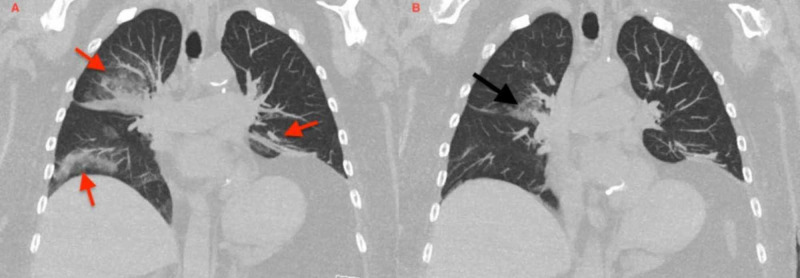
Follow-up CT Chest at three months (panel A) showed partial improvement of bilateral consolidative opacities (red arrows). Follow-up CT at six months (panel B) showing complete resolution of RUL, improved bilateral lower lobes airspace opacities, and a patchy consolidative opacity in right middle-lower lobe (black arrow) secondary to resolving ELP CT: computed tomography, RUL: right upper lobe, ELP: exogenous lipoid pneumonia
